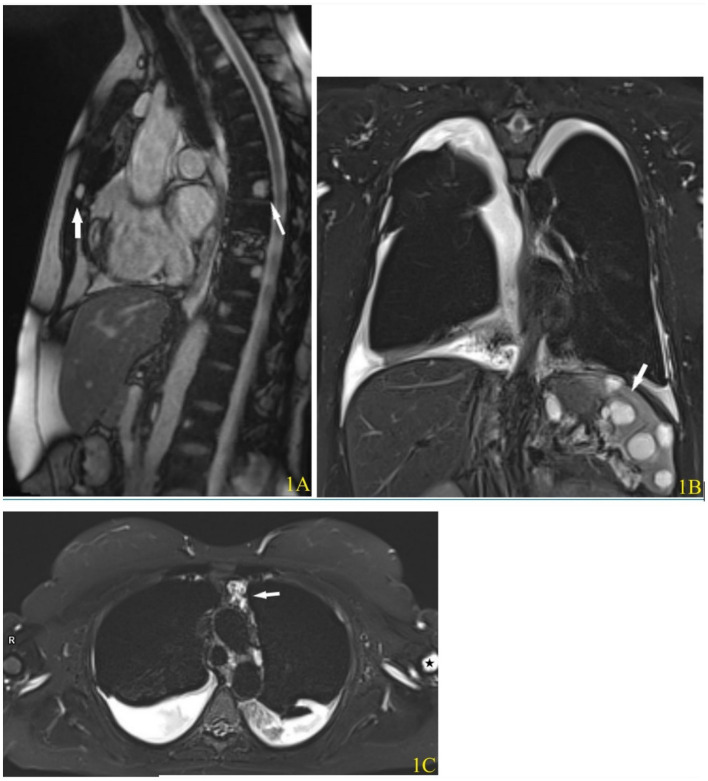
Magnetic resonance imaging of the chest of patient No. 3 at presentation. (A) T2-weighted TrueFISP image, sagittal view. Multiple lesions of T2 hyperintensity are present in the vertebral bodies and sternum (arrow). (B) T2-weighted short tau inversion recovery (STIR) image, coronal view. Multiple cystic lesions with high T2 signal are visible in the spleen (arrow). (C) T2-weighted STIR image, axial view. Bilateral pleural effusion and a bright-signal lymphatic malformation are present in the mediastinum (arrow). A hyperintense bone lesion is also present in the left humerus is also present (asterisk).
